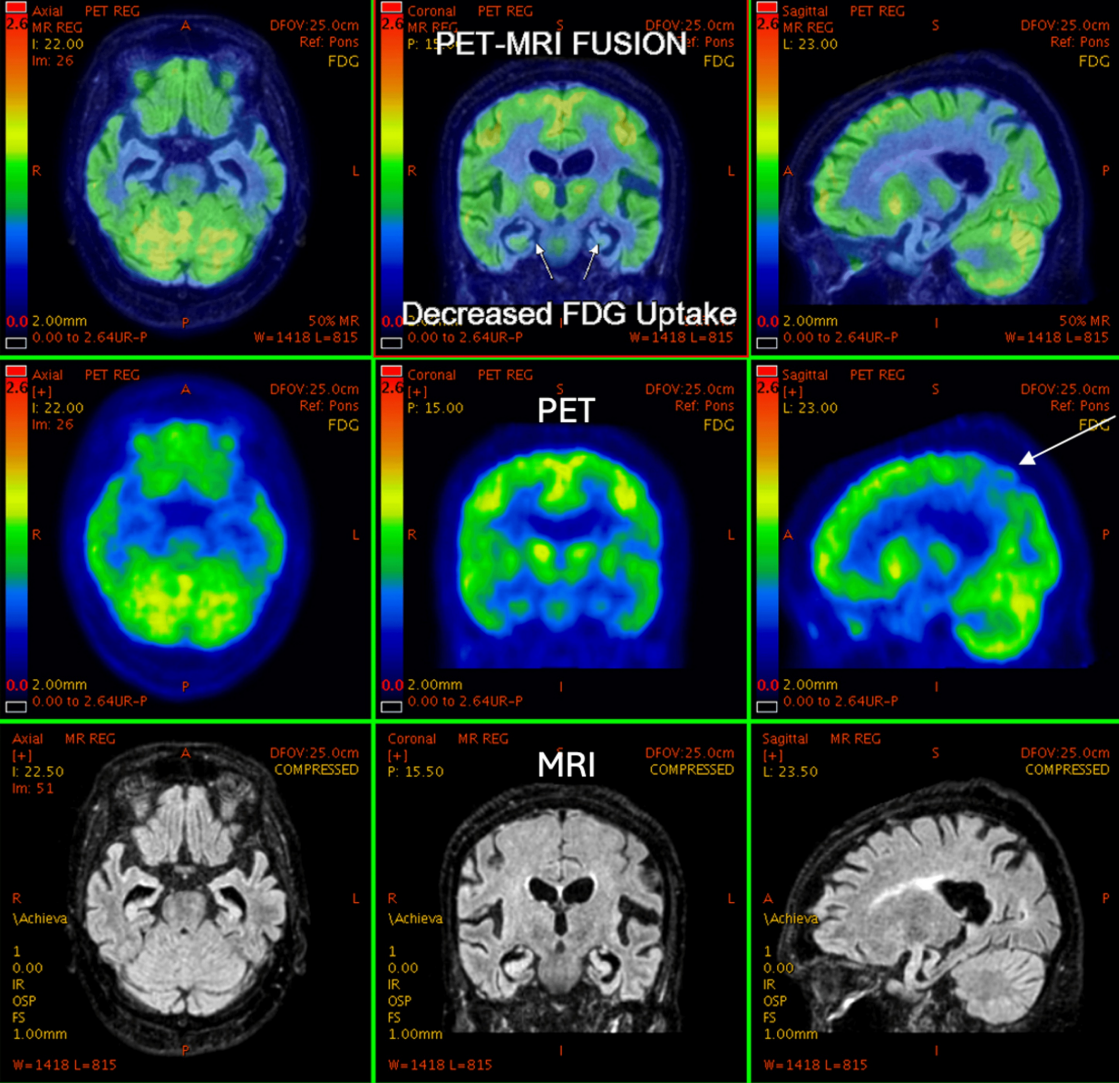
68-year-old female with a history of dementia, suspiciousness, and imbalance while doing day-to-day work for two years. Symptoms worsened for two months. PET-MRI brain study shows bilateral temporal lobes (predominantly mesial temporal lobe) and bilateral parietal lobes. Posterior cingulate gyrus shows diffuse atrophy and a symmetrical significant reduction in FDG uptake, suggesting temporoparietal pattern dementia - likely to be Alzheimer’s disease. PET: positron emission tomography; MRI: magnetic resonance imaging; FDG: fluorodeoxyglucose
